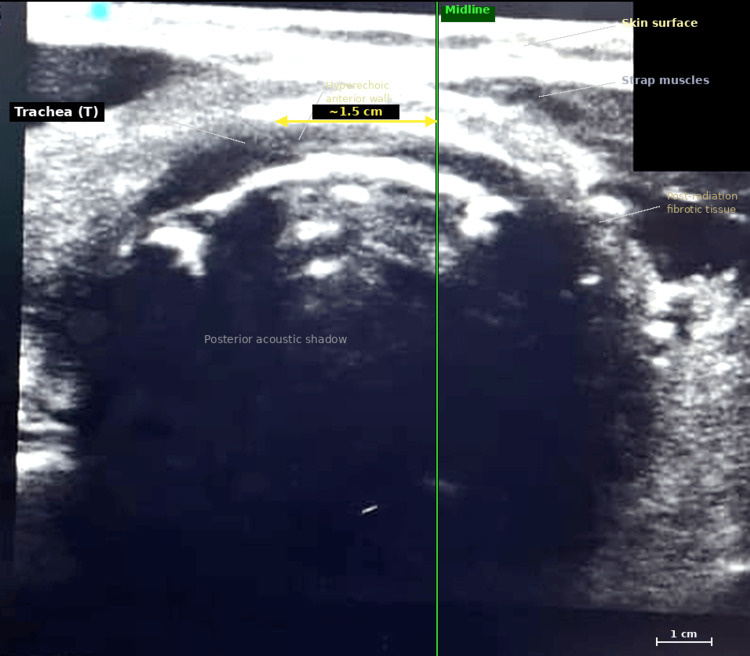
Preoperative transverse neck ultrasonography performed with a high-frequency linear probe (6-13 MHz; SonoSite M-Turbo, Fujifilm, USA) at the level of the cricoid cartilage demonstrating leftward tracheal displacement in the reported patient The trachea (T), identified by its hyperechoic anterior wall arc and characteristic posterior acoustic shadowing from the air-filled lumen, is displaced approximately 1.5 cm to the left of the anatomical midline (green vertical line). The surrounding heterogeneous echogenic tissue reflects post-radiation fibrosis of the cervical soft tissues. The strap muscles (SM) are visible superficially beneath the skin surface (S). Date and time of acquisition have been digitally obscured to protect patient confidentiality.
